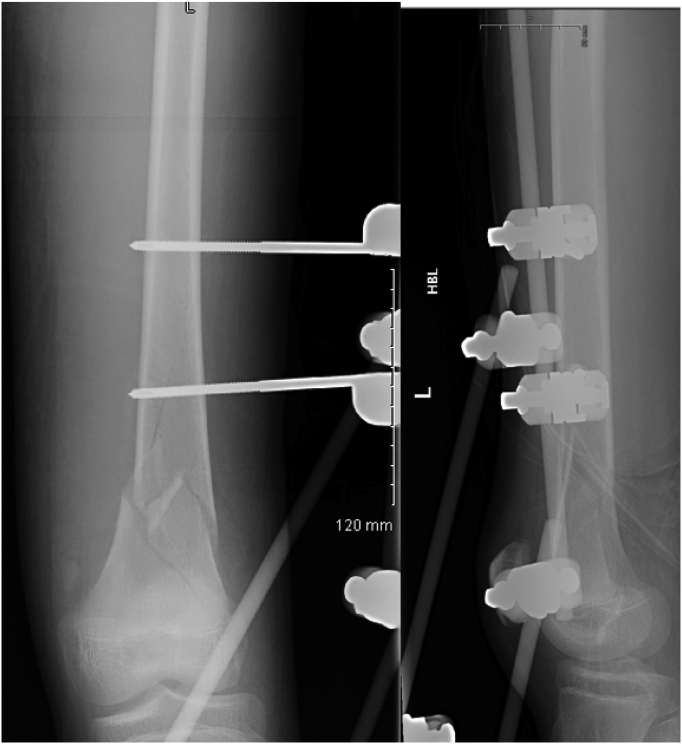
post-operative radiograph of the left distal femur with external fixator in situe.
